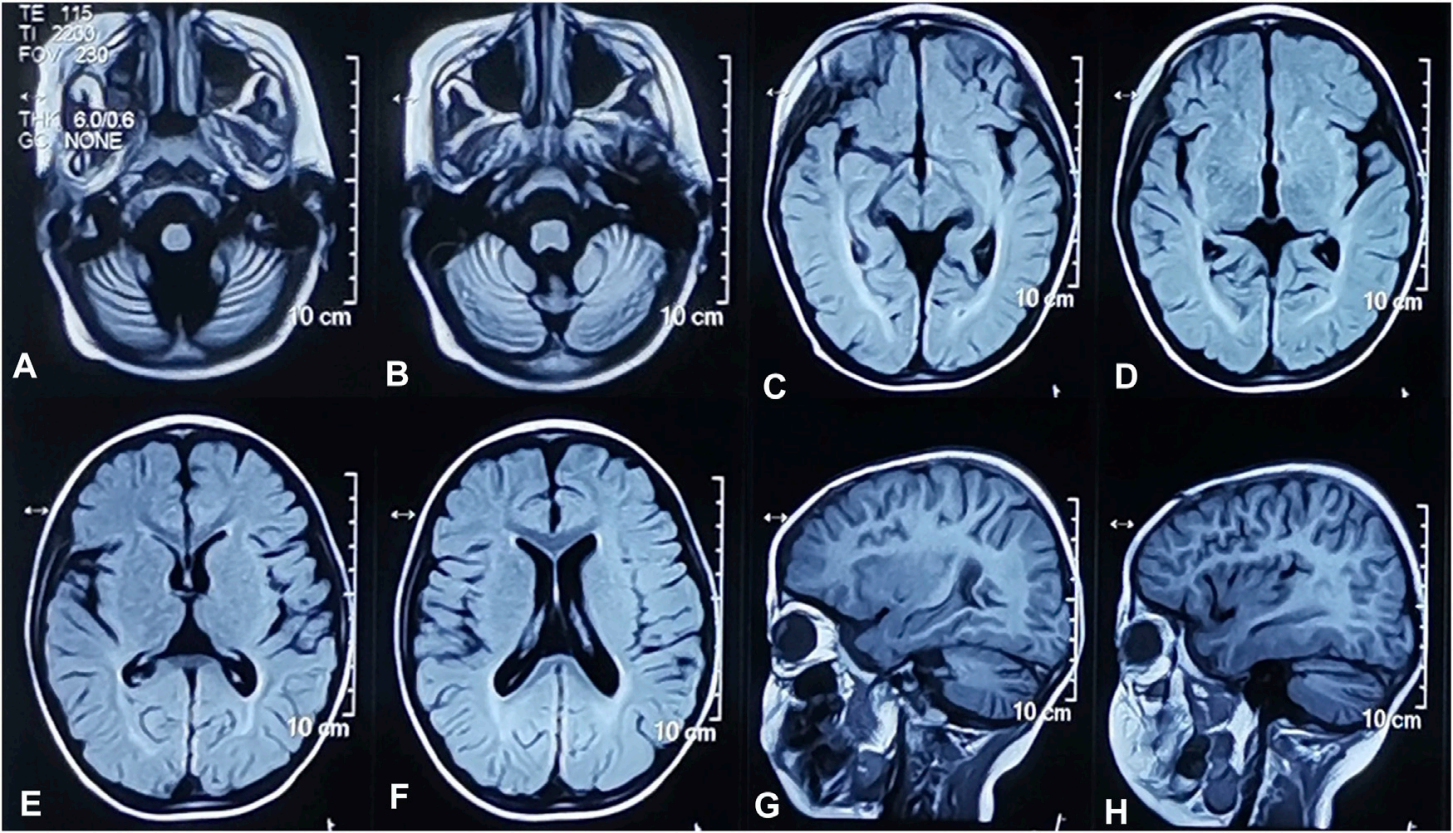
Brain MRI of the proband. Widened cerebellum sulci. Axial T2-weighted FLAIR image (A,B). Abnormal signals of white matter near the posterior horn of bilateral lateral ventricles. Axial T2-weighted FLAIR images (C,D). Widened bilateral cerebral hemispheres sulci. Axial T2-weighted FLAIR image (E,F). Widened bilateral cerebral hemispheres and cerebellar sulci. Sagittal T1-weighted images (G,H).
